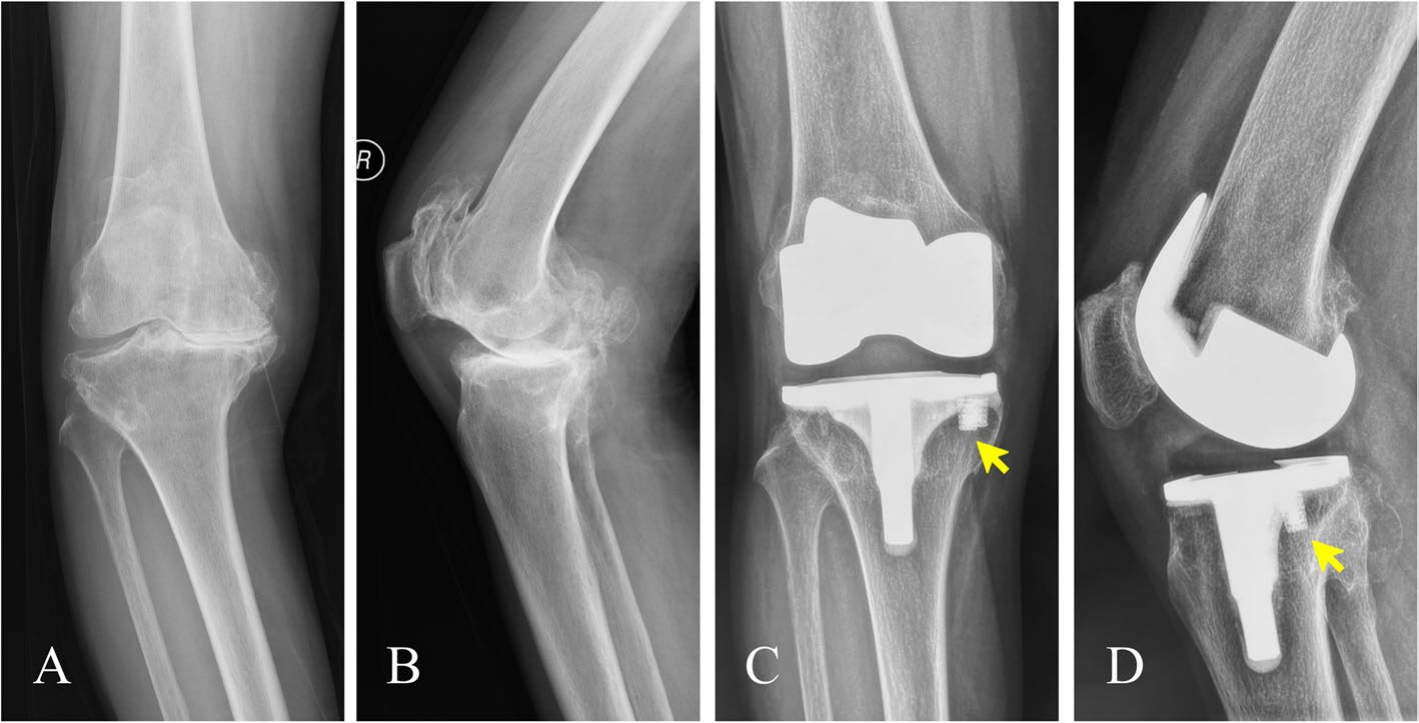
Preoperative and postoperative radiographs of a 64-year-old female patient. A Osteoarthritis was evidenced on the preoperative anteroposterior radiograph. B Osteoarthritis was evidenced on the preoperative lateral radiograph. C Trabecular bone formation (yellow arrow) around the pillar was noted on the anteroposterior radiograph 2.5 years after surgery. D Trabecular bone formation (yellow arrow) around the pillar was noted on the lateral radiograph 2.5 years after surgery
